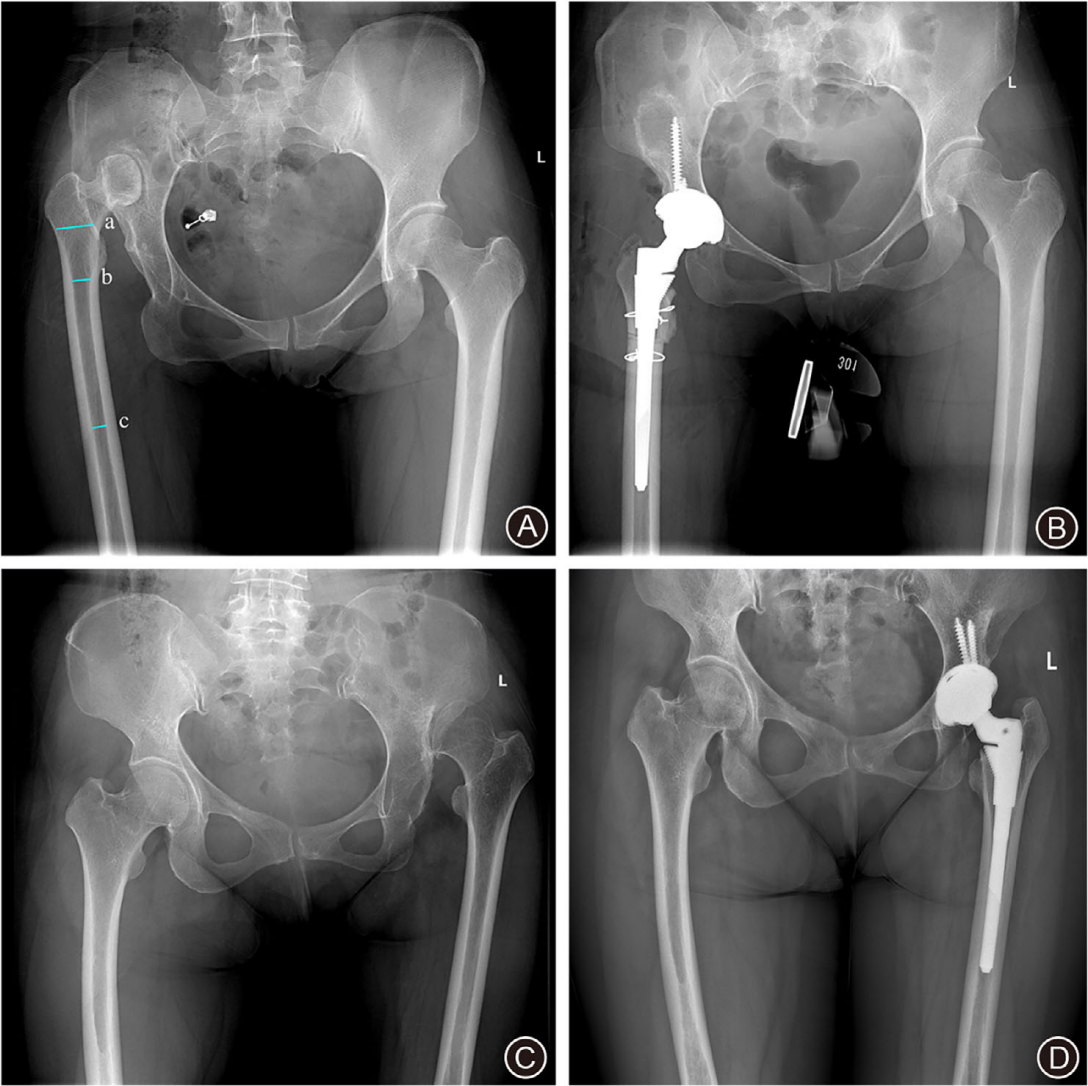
A 36‐year‐old female with right Crowe type IV DDH (A and B) and a 50‐year‐old female with left Crowe type IV DDH (C and D). (A) The preoperative AP hip radiograph (CFI: 2.81, MCFI: 2.44, DCFI: 1.15). (B) The postoperative radiograph 2 days after right hip surgery. THA with STO was performed. (C) The preoperative AP hip radiograph (CFI: 4.25, MCFI: 2.24, DCFI: 1.89). (D) The postoperative radiograph 2 days after right hip surgery. THA without STO was performed. a: 20 mm above the center of lesser trochanter (CLT), b: 20 mm below the CLT, c: isthmus.
